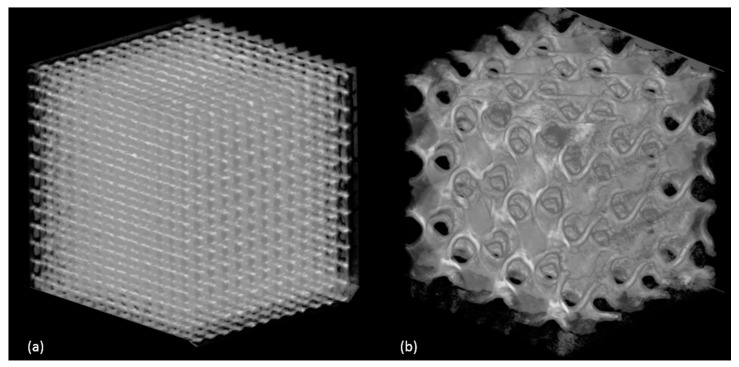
CT reconstruction of samples: (a) cube; and (b) gyroid.
